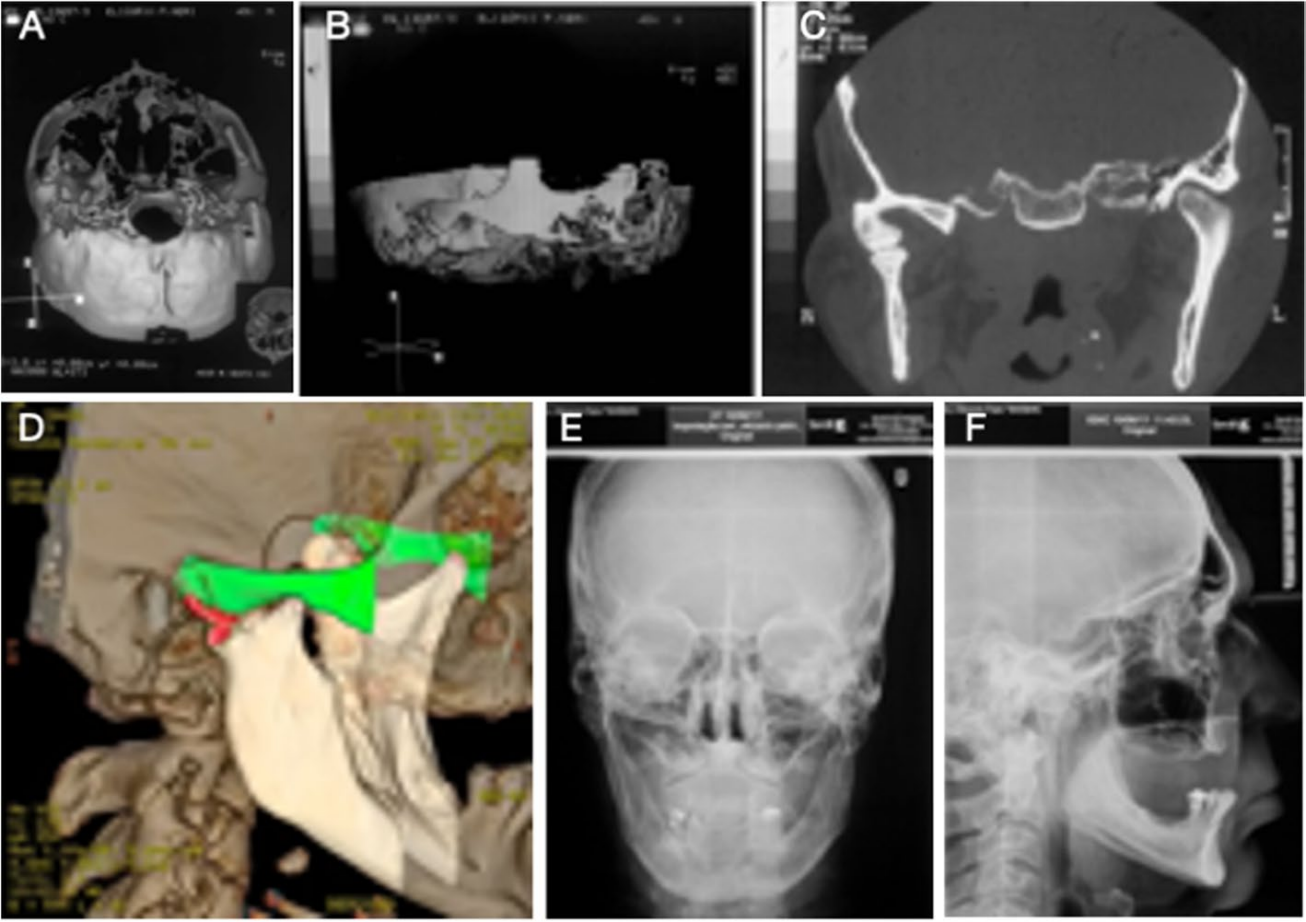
Postoperative imaging. A, B and C Computed tomography (17-year follow-up). The presence of poly(methyl methacrylate) (PMMA) components used in the Puricelli biconvex arthroplasty technique is observed on the right side. The components are stabilised in the initial position, near the base of the skull and the condylar region of the mandible. D Computed tomography with 3D reconstruction (39-year follow-up). Stability of the prosthetic components is observed, with no surgical reintervention during this period. E and F Frontal and lateral radiography, respectively (39-year follow-up). The facial skeletal relationship shows normal patterns
